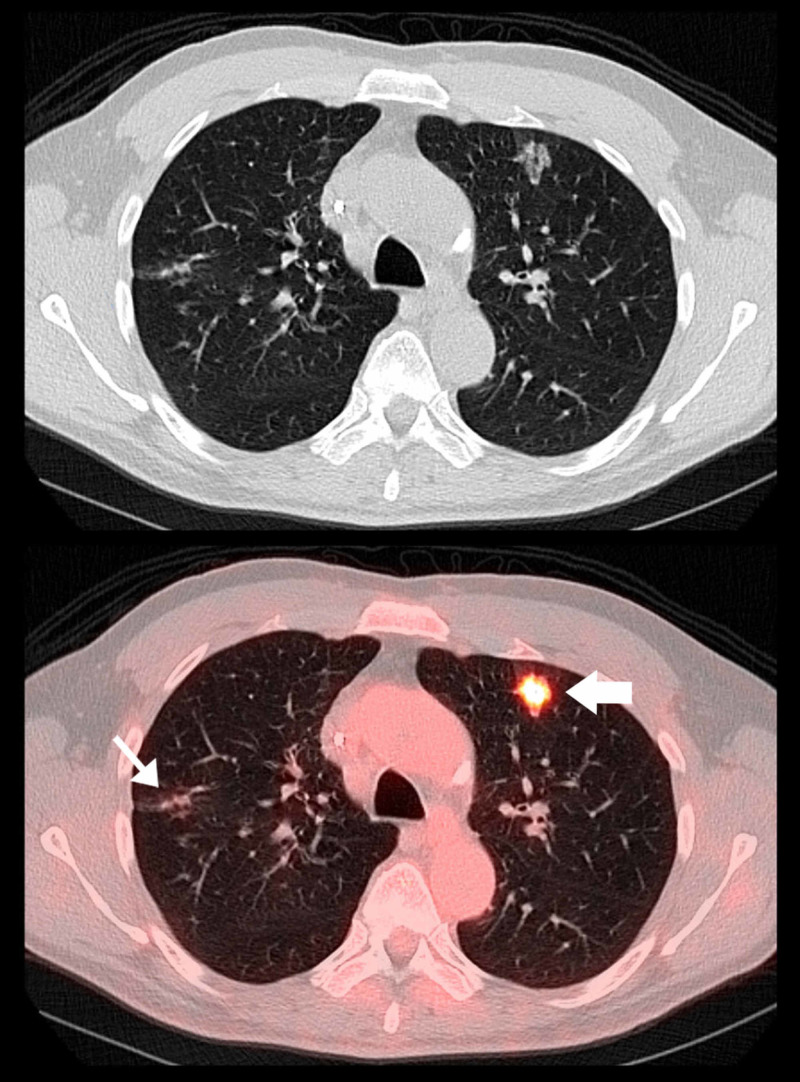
Selected axial CT and axial PET-CT fusion images. Selected axial CT and axial PET-CT fusion images through the mid chest level in lung windows demonstrate a peripheral, rounded ground-glass opacity with reticular characteristics and an air bronchogram in the left upper lung lobe (wide arrow). In the right upper lobe, a reticular ground glass opacity is also seen (thin arrow). The lesions also demonstrated increased fluorodeoxyglucose (FDG) uptake measuring up to standardized uptake value (SUV) 6.7.
